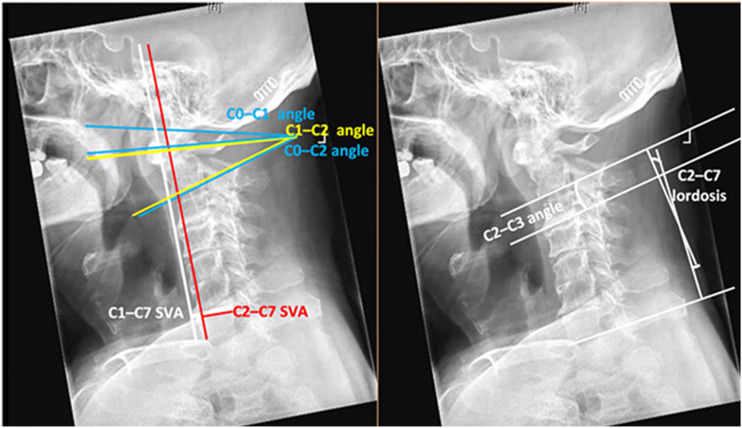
Sagittal radiographs showing the parameters for cervical alignment.
